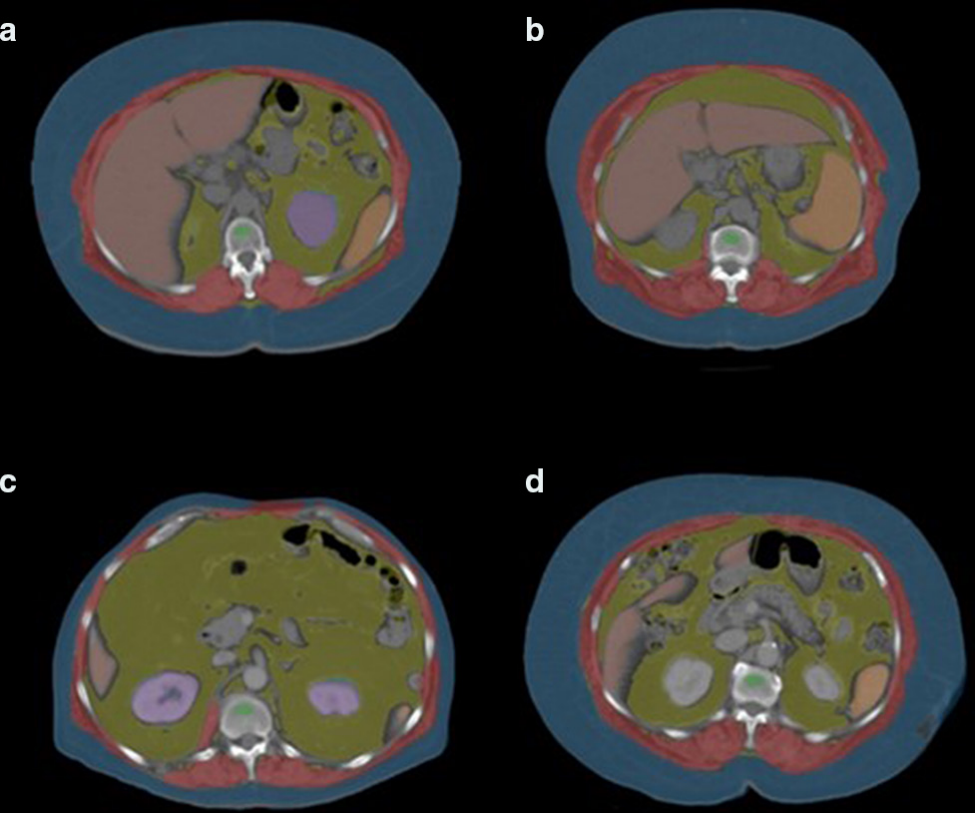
Non-contrast transverse (axial) CT images at the L1-level superimposed with AI-based fully automated tissue segmentation in four fall cases with abnormal muscle, bone, and fat measures relative to the 80% specificity thresholds (Table 4). (a) 58-year-old female, abdominal CT for urolithiasis. Presented 19 years later with a fall after her legs gave out while standing and multiple rib fractures. Abnormal L1-level measures for TAT (659.2 cm^2^), muscle attenuation (−14.1 HU), and trabecular attenuation (107.6 HU). (b) 69-year-old female, CT for recurrent UTIs. Presented 12 years later with a fall after slipping on a wet surface and distal radius fracture. Abnormal L1-level measures for TAT (648.5 cm^2^), muscle attenuation (−28.5 HU), and trabecular attenuation (92.0 HU). (c) 67-year-old male, CT after fall revealed multiple vertebral compression fractures. Abnormal L1-level measures for TAT (627.4 cm^2^), muscle attenuation (−11.5 HU), and trabecular attenuation (103.9 HU). (d) 63-year-old female, CT urography for hematuria. Presented 10 years later with a fall after tripping on stairs and multiple rib fractures. Abnormal L1-level measures for TAT (710.6 cm^2^), muscle attenuation (−23.8 HU), and trabecular attenuation (98.2 HU). For reference, the red overlay = skeletal muscle, yellow = visceral adipose tissue, blue = subcutaneous adipose tissue, and green = a region of interest in the L1 vertebral body trabecular bone. HU, Hounsfield unit; TAT, total adipose tissue.
